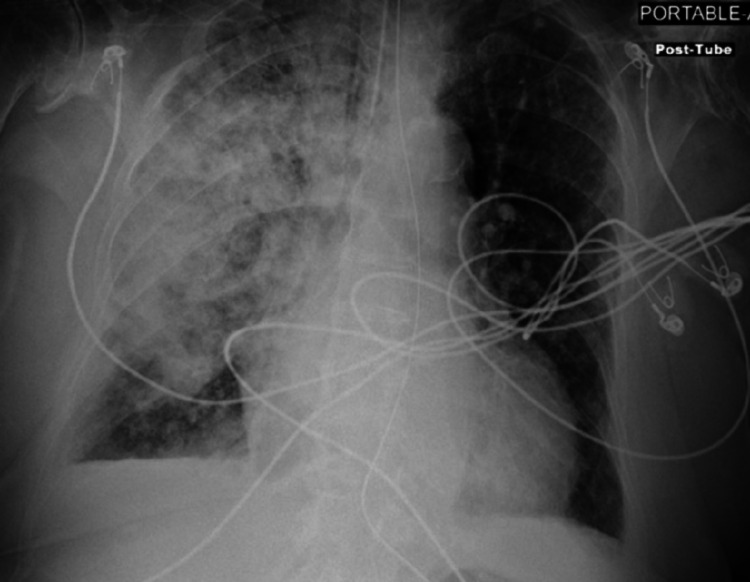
Portable chest radiograph following intubation demonstrated worsening of the right lung infiltrate. The left lung is without any significant abnormality.
